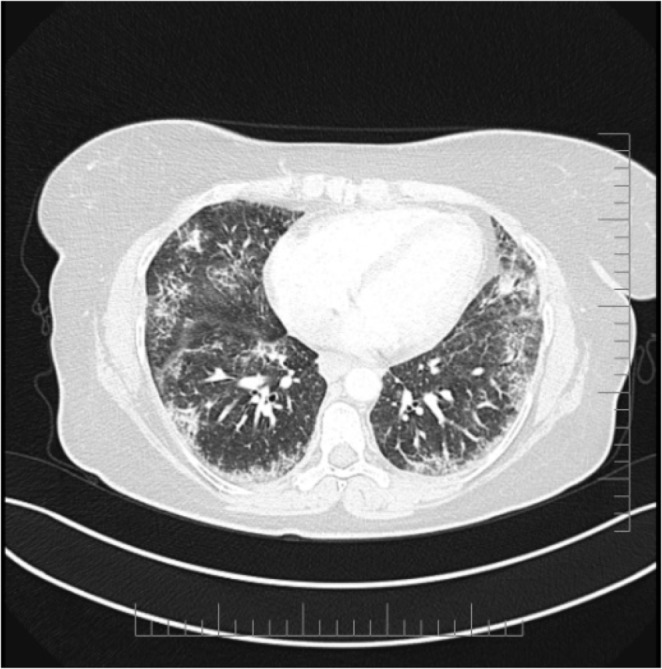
Chest CT scan showing thickened linear markings, ground glass opacities, and mild bronchiectasis.
